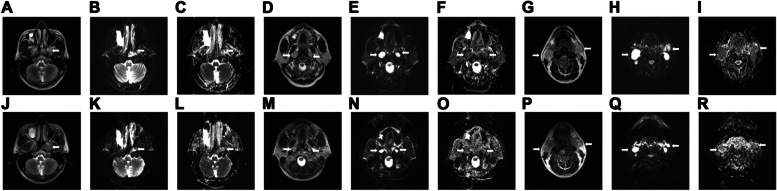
Images of a 47-year-old man with LA-NPC showing intermediate response after induction chemotherapy: (A, D, and G) primary lesion (arrow) in axial T2-weighted MRI with (B, E, and H) corresponding axial diffusion-weighted MRI with b values of 0 and 1000 s/mm^2^ (C, F, and I), apparent diffusion coefficient (ADC) maps pretreatment, and (J-R) matched sections of the same imaging sequences after induction chemotherapy
